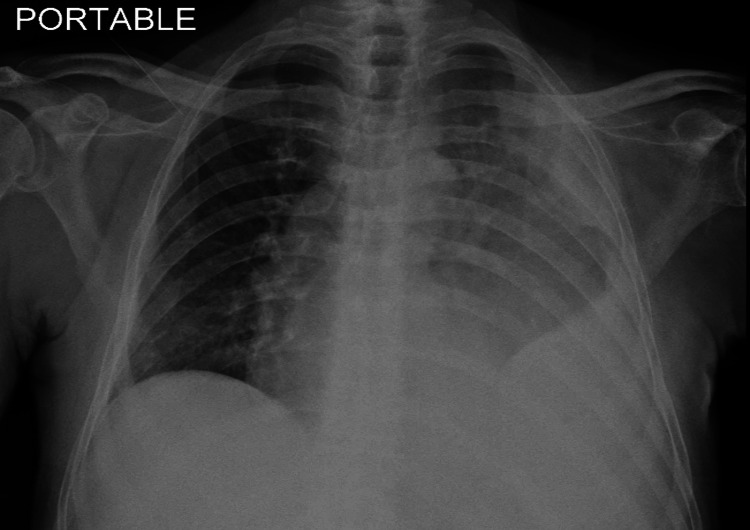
Initial chest-X-ray showing infiltrates in the left lung with moderately large left pleural effusion
